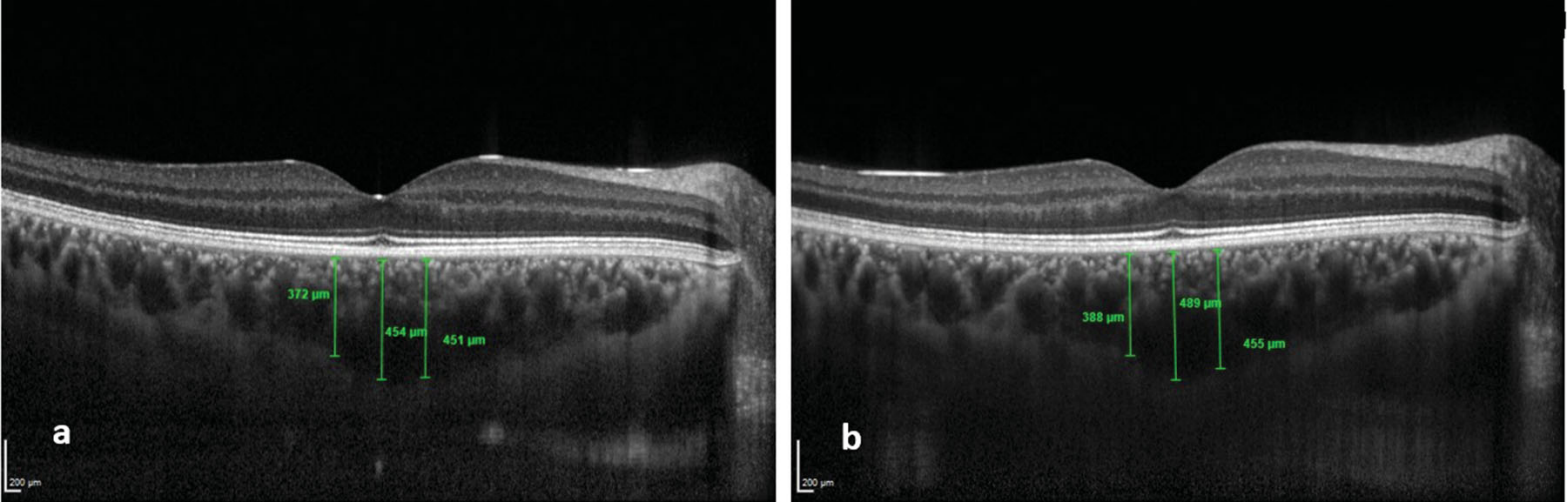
CT measurements using enhanced depth imaging optical coherence. (a) CT measured subfoveally and 500 µm nasal and temporal to the fovea before HC exposure. (b) Increased CT in the same eye after HC exposure.
